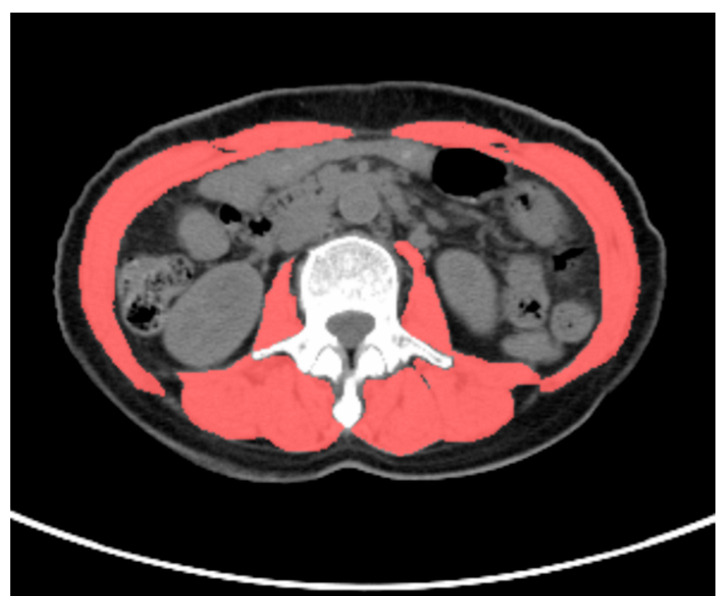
Methodology for measuring skeletal muscle cross-sectional area. A 58-year-old female patient with gastric cancer underwent preoperative ^18^F-FDG PET/CT examination. The total skeletal muscle cross-sectional area at the L3 vertebral level was delineated based on a CT attenuation range (−29 HU to 150 HU (scarlet color )) on CT images using LIFEx software version 7.4.0. The total skeletal muscle cross-sectional area at the L3 vertebral level in this patient was 91.2 cm^2^.
